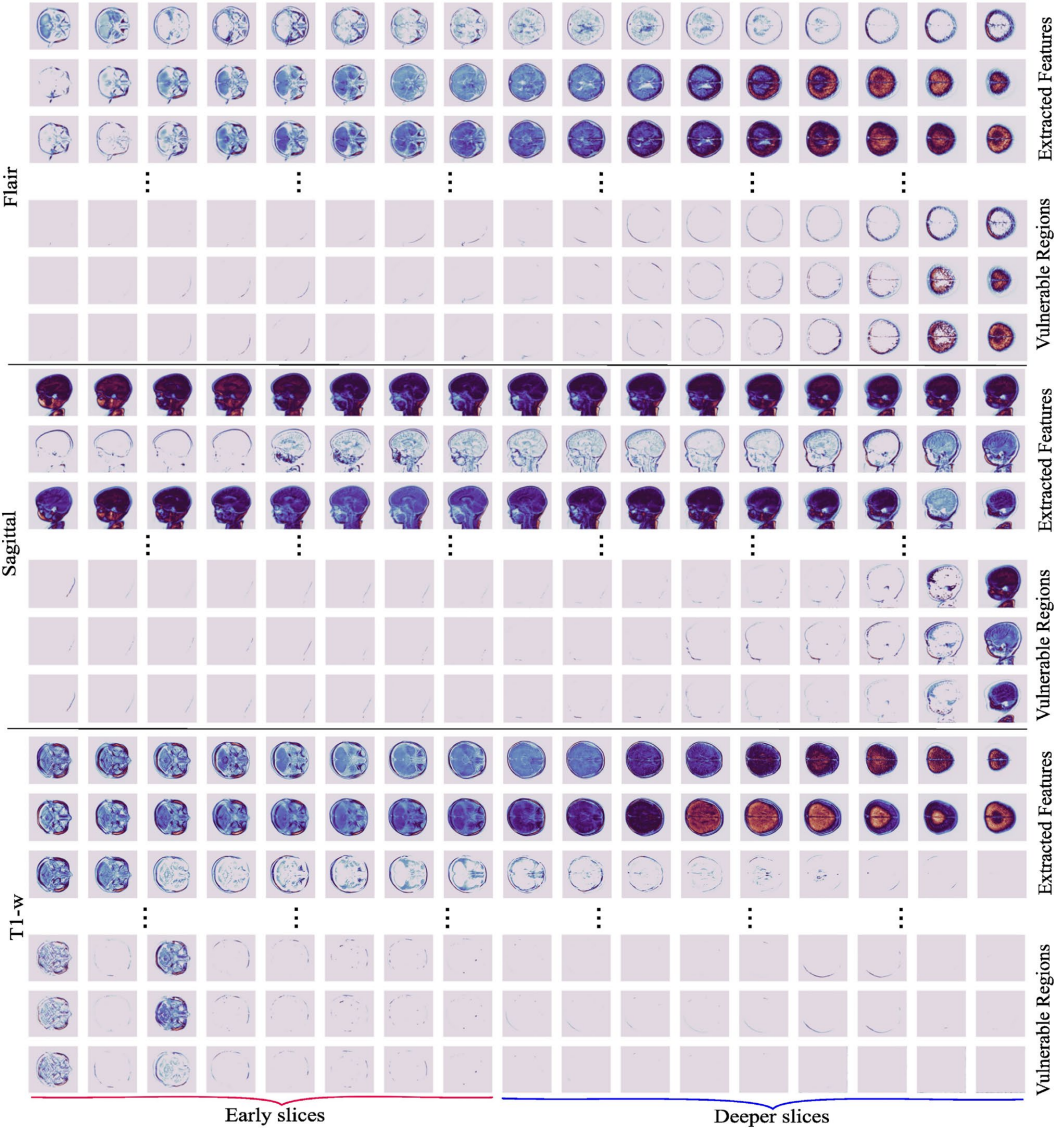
The three MRI scans, including Flair, Sag, and T1-w, have been depicted with unique learning trends. In each MRI scan, the first row represents generalized captured features by the DL model, while the second row shows the focused or salient feature maps
